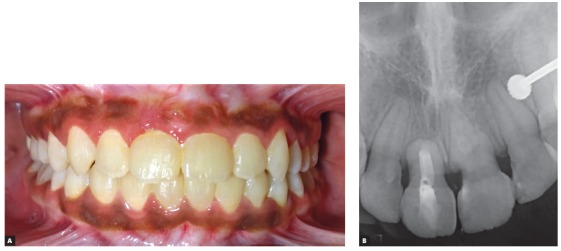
A) Intraoral frontal view at 3-year follow up. B) Periapical radiograph at 3-year follow up.
